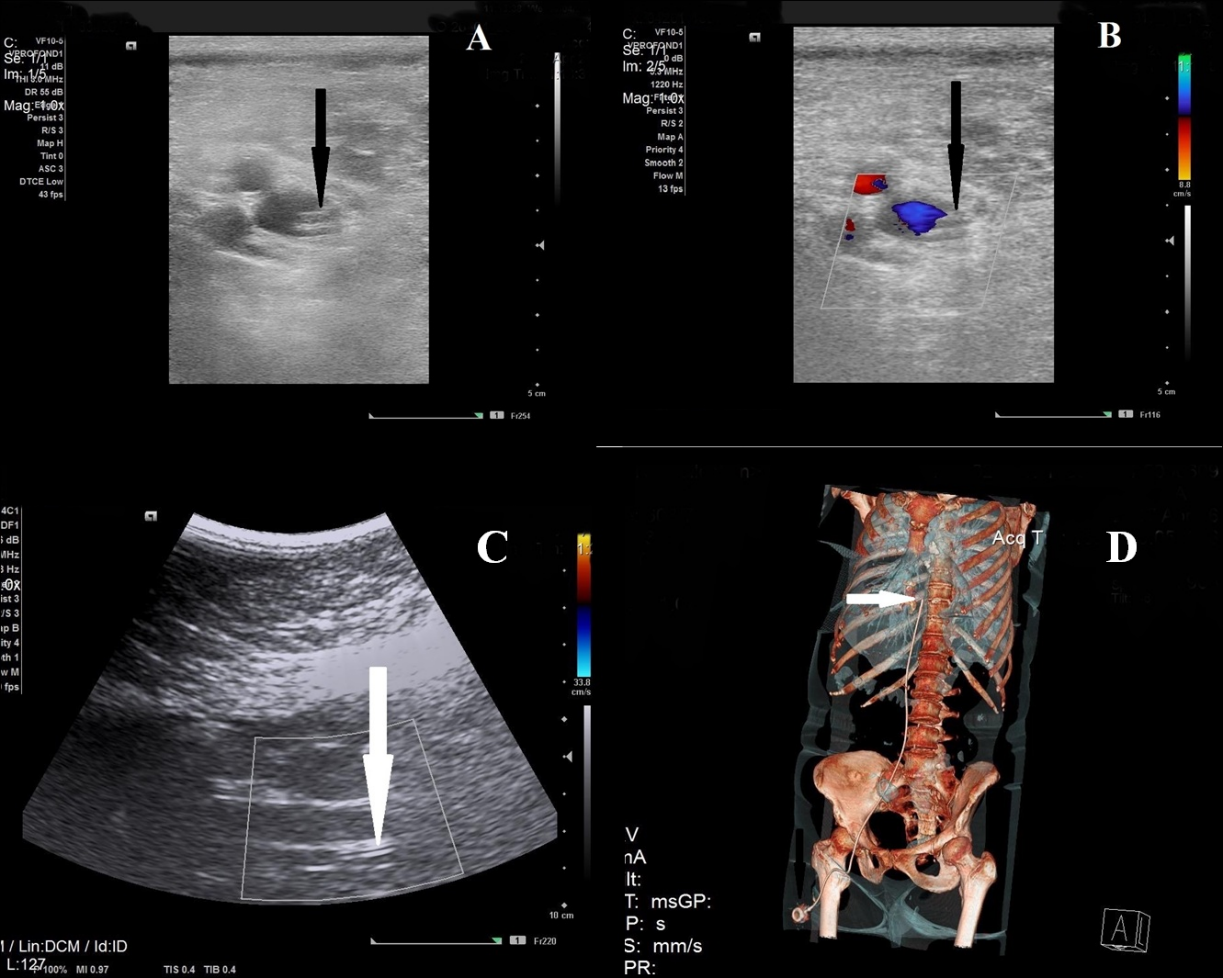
Femoral port-system vizualisation by different imaging modalities A. Ultrasound scan. Saphenofemoral confluence. B-mode, adequate catheter position (arrow). B. Ultrasound scan. Saphenofemoral confluence. Color Doppler, adequate catheter position (arrow). C. Transabdominal ultrasound scan. Inferior caval vein. Adequate catheter position (arrow). D. Whole body CT scan. TIVAP capsule on the right hip, catheter tip in the proximal part of IVC close to heart right atrium (white arrow). Abbreviations: CT, computed tomography; TIVAP, totally implanted venous access port; IVC, inferior vena cava.
